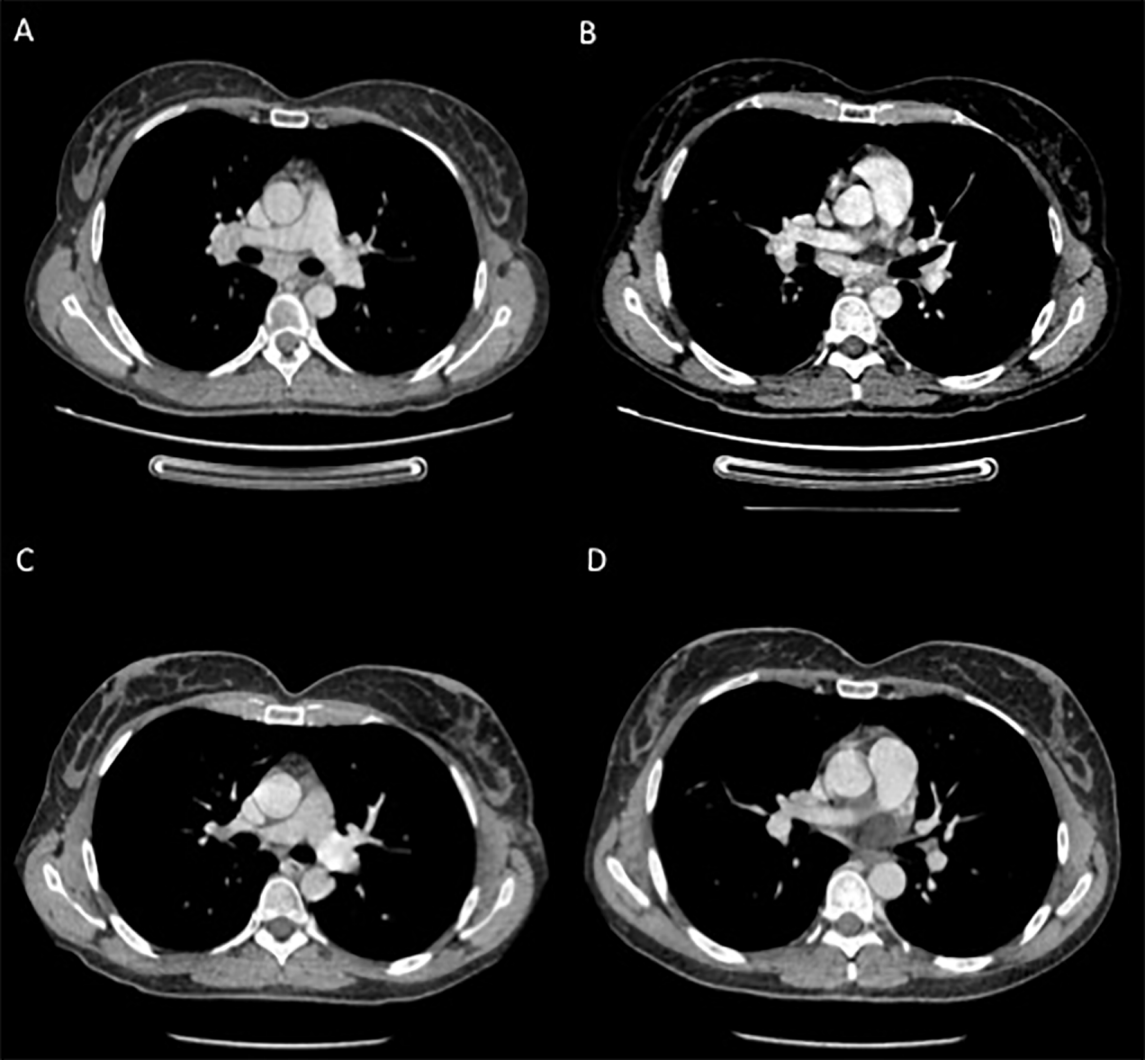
Contrast CT-scans performed during targeted therapy (A, B) showing enlarged mediastinal lymph nodes; and (C, D) after the definitive suspension of treatment showing reduction in the diameter of the previously reported lymph nodes.
